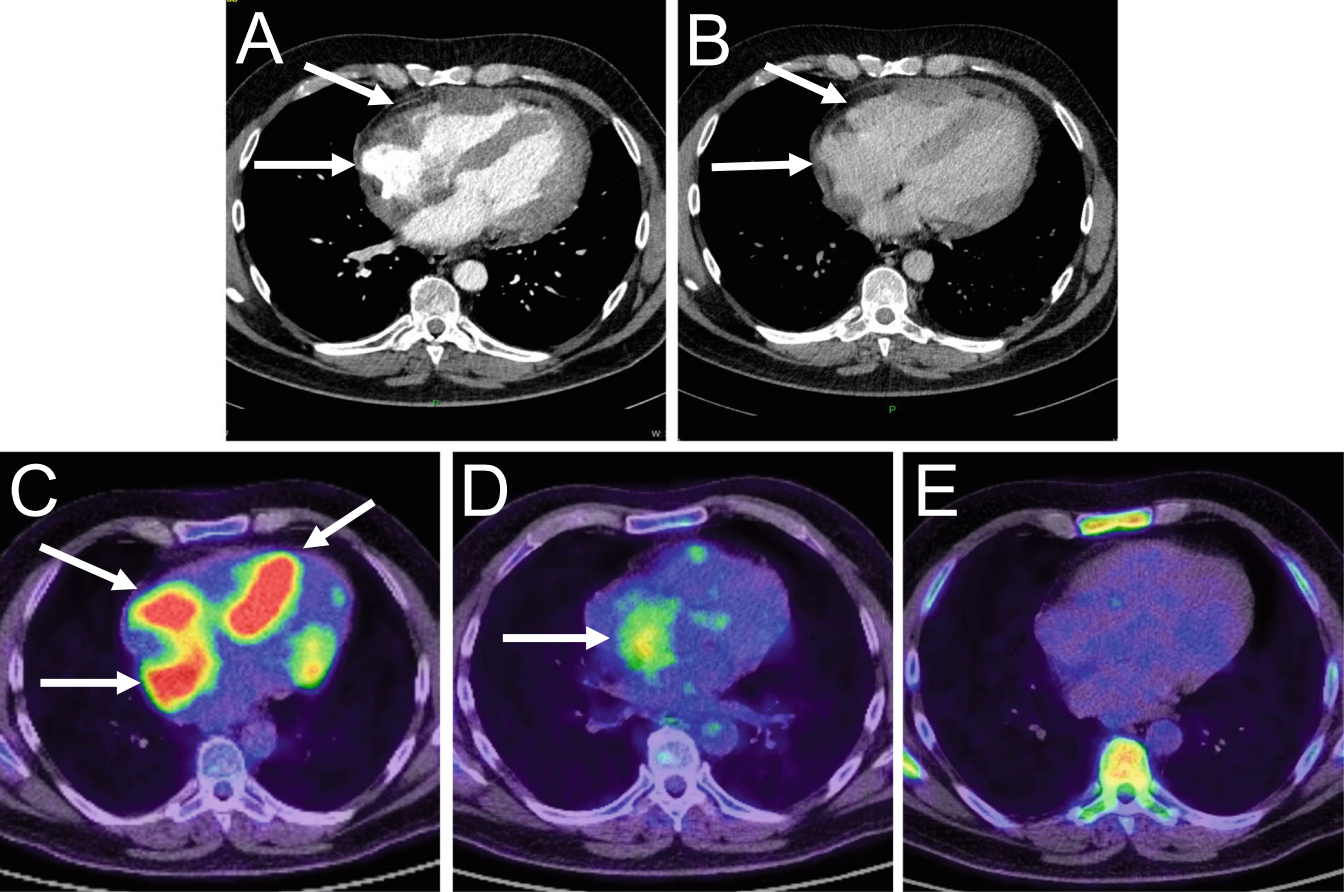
Chest CT and PET/CT images of the patient. a, b Contrast-enhanced chest CT transaxial reconstruction shows the inhomogeneous hypodense tissue in the right atrium and ventricle and circumferential pericardial effusion (arrows). c–e Fused 18-fluorodeoxyglucose (FDG) PET/CT image axial sections demonstrate the right atrium and ventricle mass along with the FDG uptake within the tumor (arrows), the images were made before (c), during (d), and after (e) the patient received chemotherapy to treat his intramyocardial metastasis
